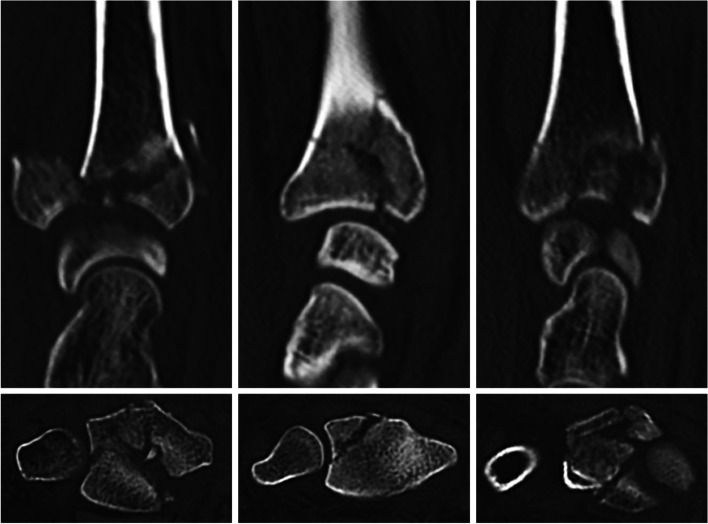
Coronal (top) and axial (bottom) computed tomography scans of clinical cases with intraarticular distal radius fractures with different degree of lunate facet comminution comprising two fragments of a comparable size (left), a smaller dorsal and a larger volar fragment (middle), and three fragments of a comparable size (right)
